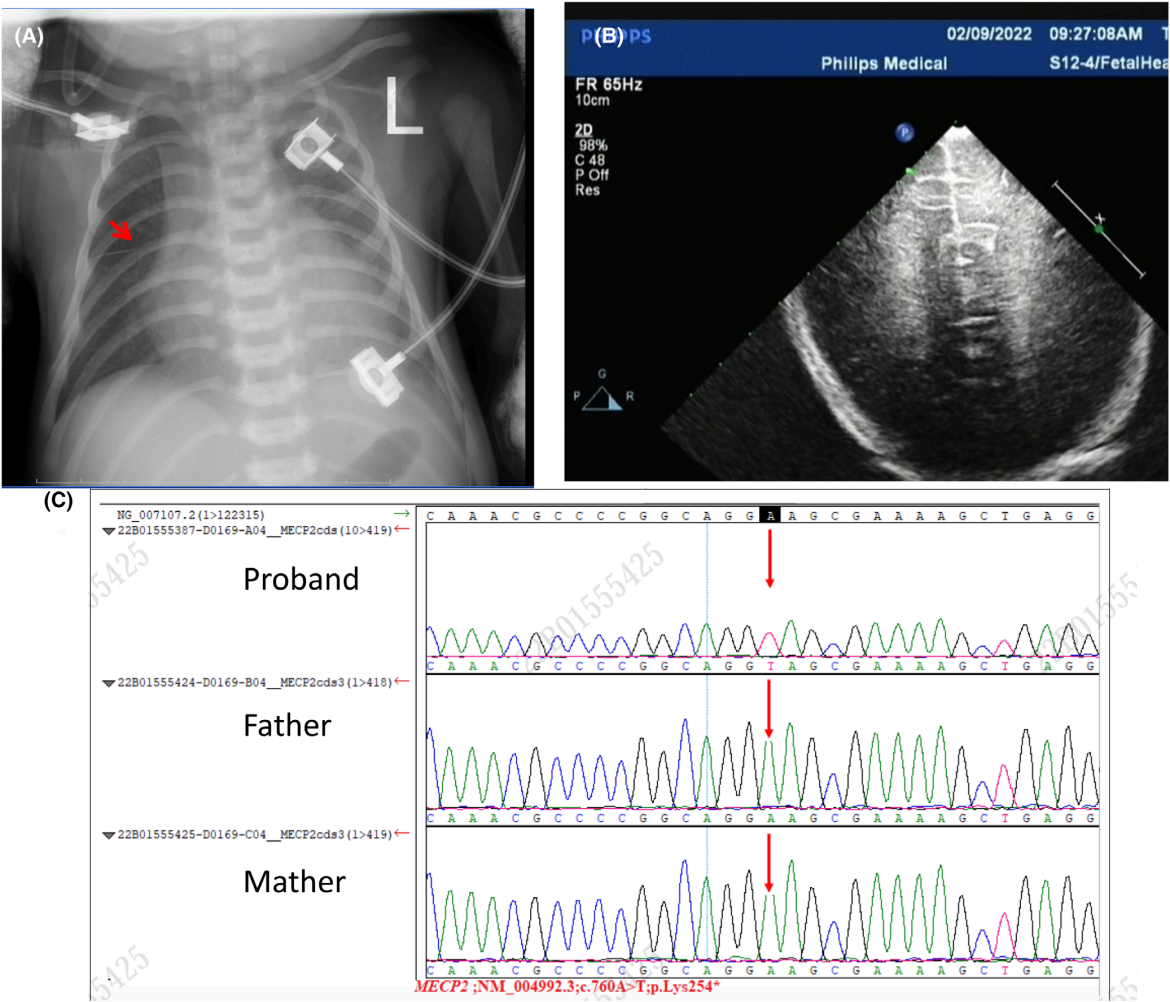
Phenotype of the proband. (A) D2 Chest x‐ray shows that the transparency of both lungs is slightly low; the markings of both lower lungs are blurred with horizontal fissures (red arrow). (B) Echocardiography shows that the foramen ovale is shunted from left to right (2.8 mm) with mild mitral and tricuspid regurgitation in the proband. (C) First‐generation sequencing verifies disease‐causing genes in the proband and family members: The MeCP2 p.Lys254* variant.
